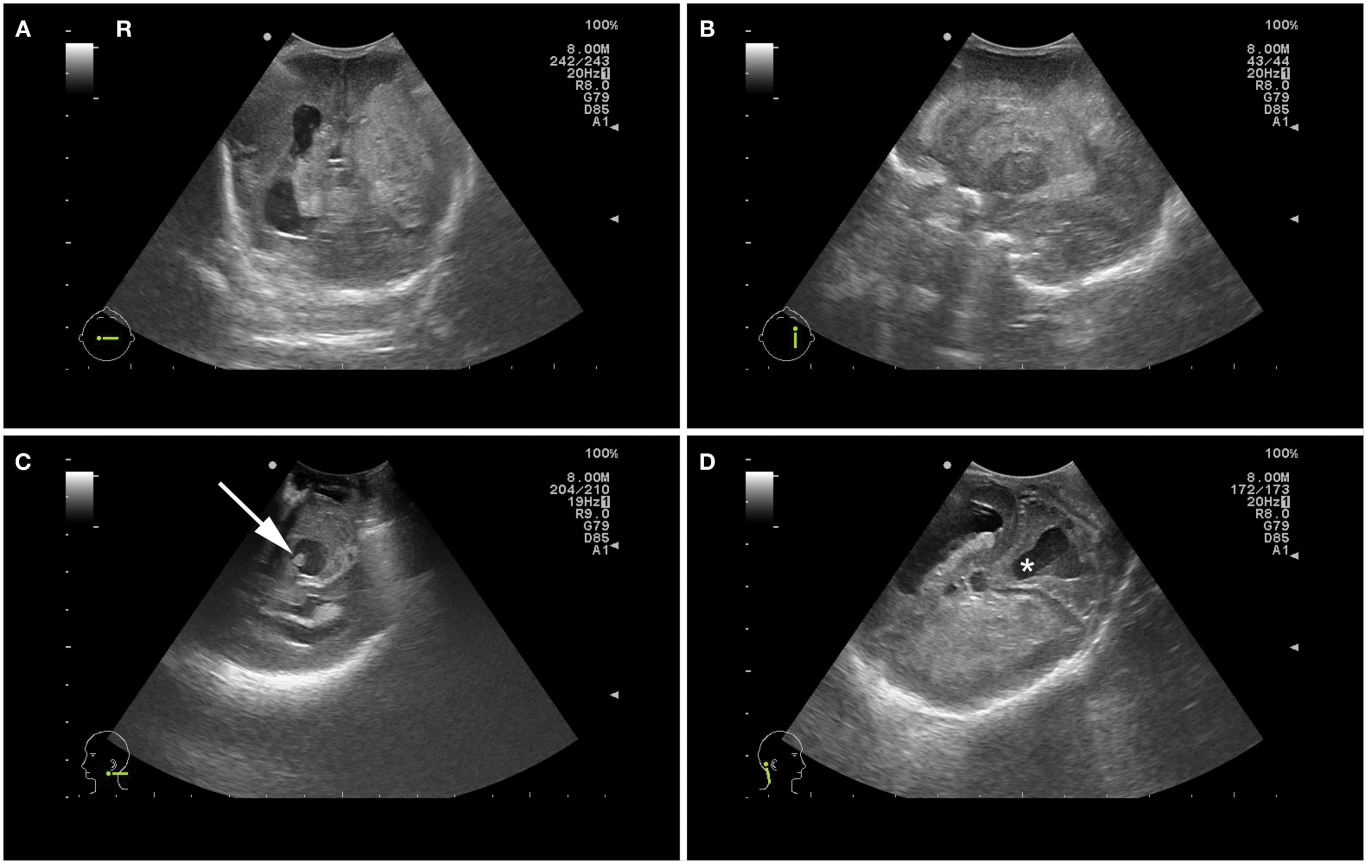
Postnatal cranial ultrasound images showing bilateral intraventricular hemorrhage with extensive hemorrhagic infarction of the left hemisphere and ventricular dilatation in coronal view (A) and parasagittal left plane view (B), blood clot in the 4^th^ ventricle (C) and dilatation of the 4^th^ ventricle (*) with dysplastic cerebellar hemispheres (D).
